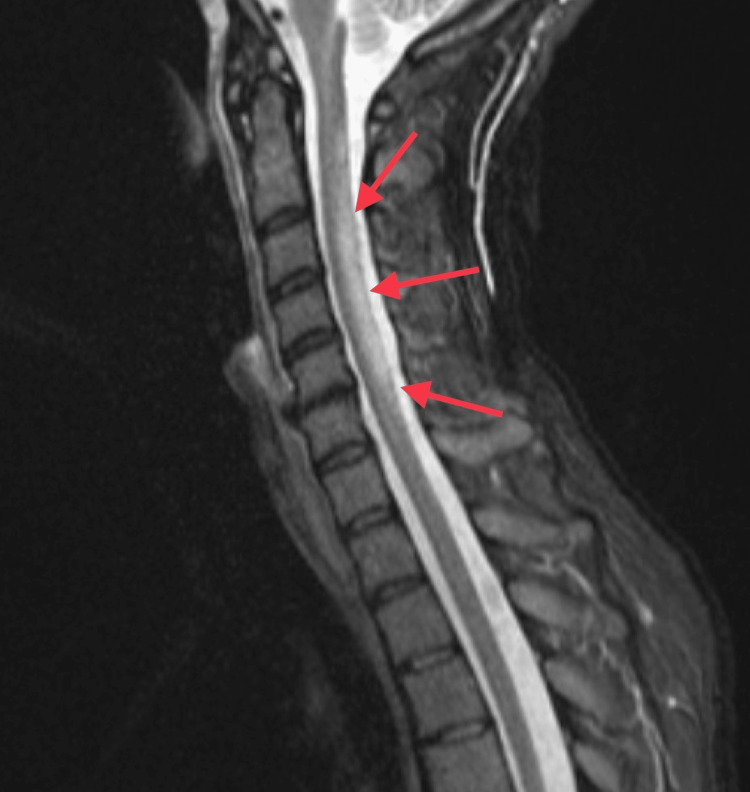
MRI showing T2 hyperintensity involving the dorsal columns of C2-C6
